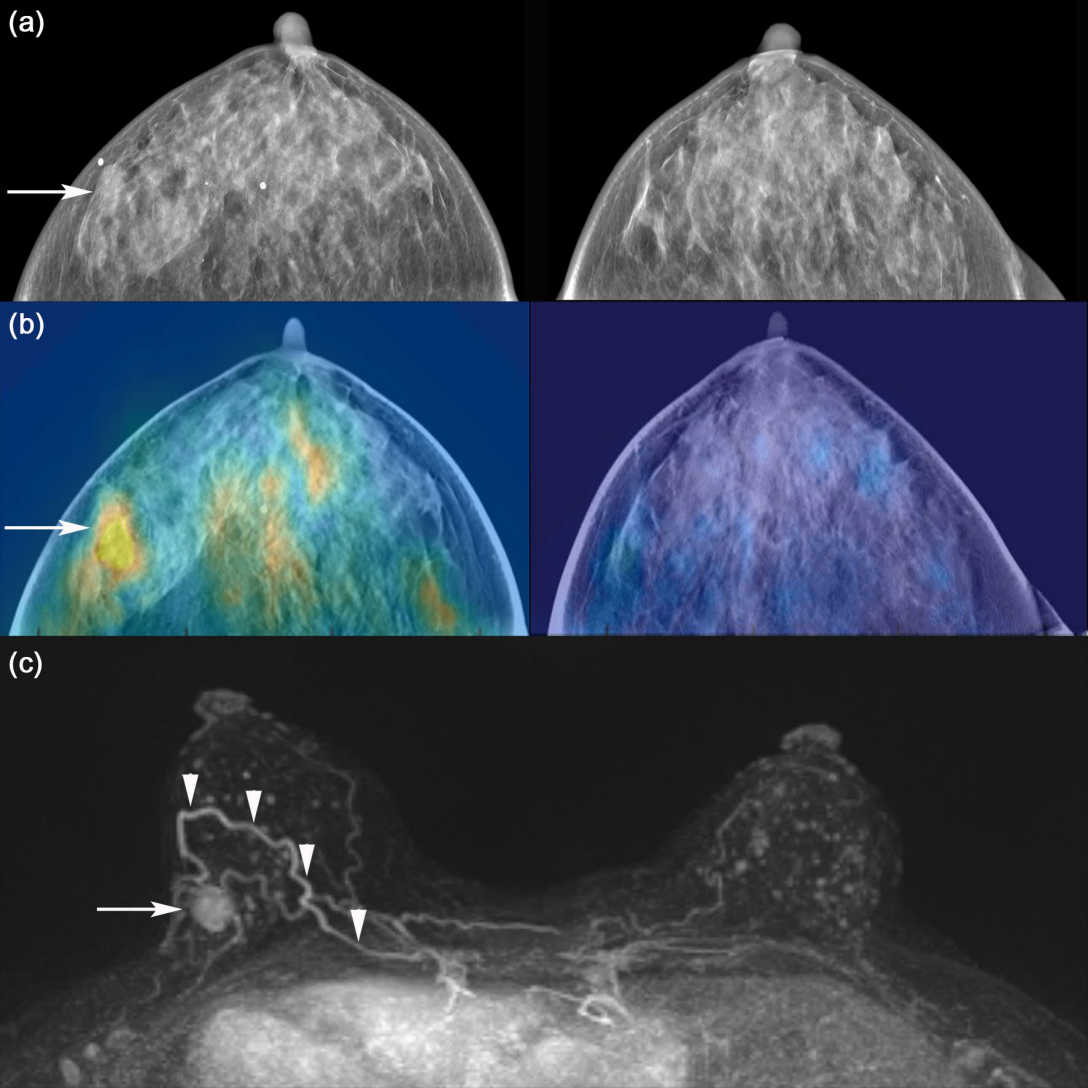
A 52-year-old woman with an invasive ductal carcinoma in the right breast. DBT images (a) show an irregular shaped mass (arrow) in the right outer breast. Reconstructed DBT/DOT fusion images (b) show asymmetrically increased activity (arrow) in the corresponding area of the right breast. On breast magnetic resonance imaging (MRI) (c), an enhancing mass (arrow) with heterogeneous enhancement is seen on maximum intensity projection (MIP) image. A dominant feeding vessel (arrowheads) with its origin in the internal mammary artery is also clearly visible. Surgery confirmed invasive ductal carcinoma.
